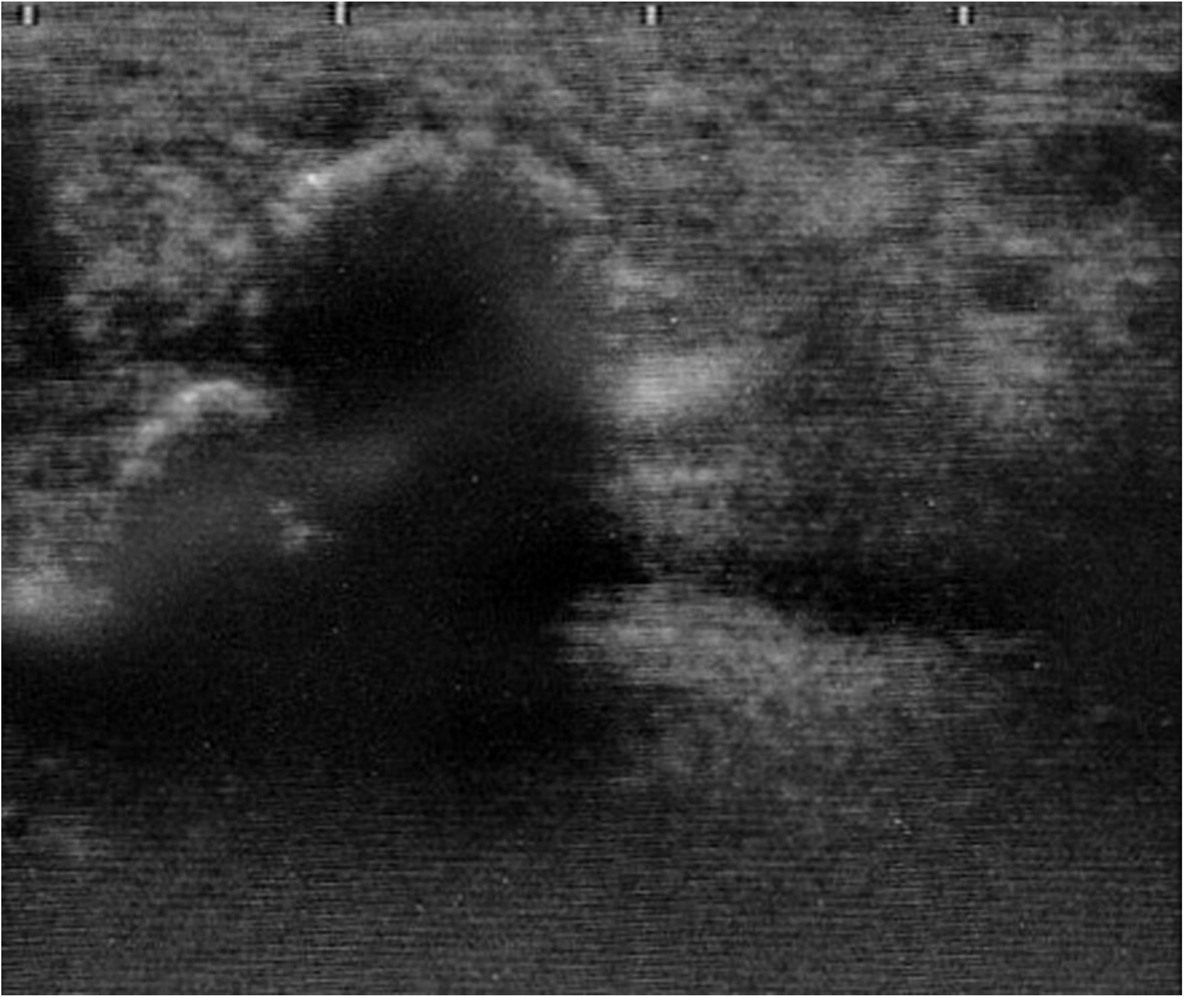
Ultrasound image of the hand. A lesion with a firm surface was present.
